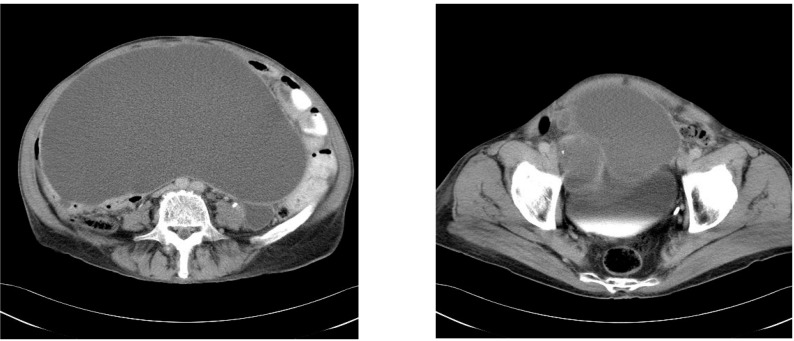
CT images –Large abdominal tumoral formation extending to the vesical cap
